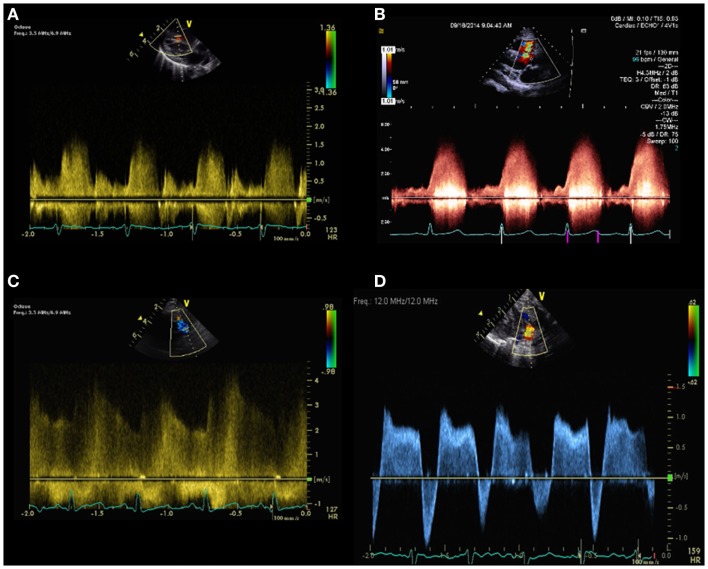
Spectral Doppler pattern across a ventricular septal defect with low velocity left to right shunt (A) indicating pulmonary hypertension and high velocity left to right shunt (B) indicating low pulmonary pressure. Spectral Doppler pattern across patent ductus arteriosus with continuous high velocity left to right shunt (C) indicating lower pulmonary artery pressure compared to aortic pressure and bidirectional shunt (D), indicating pulmonary hypertension.
